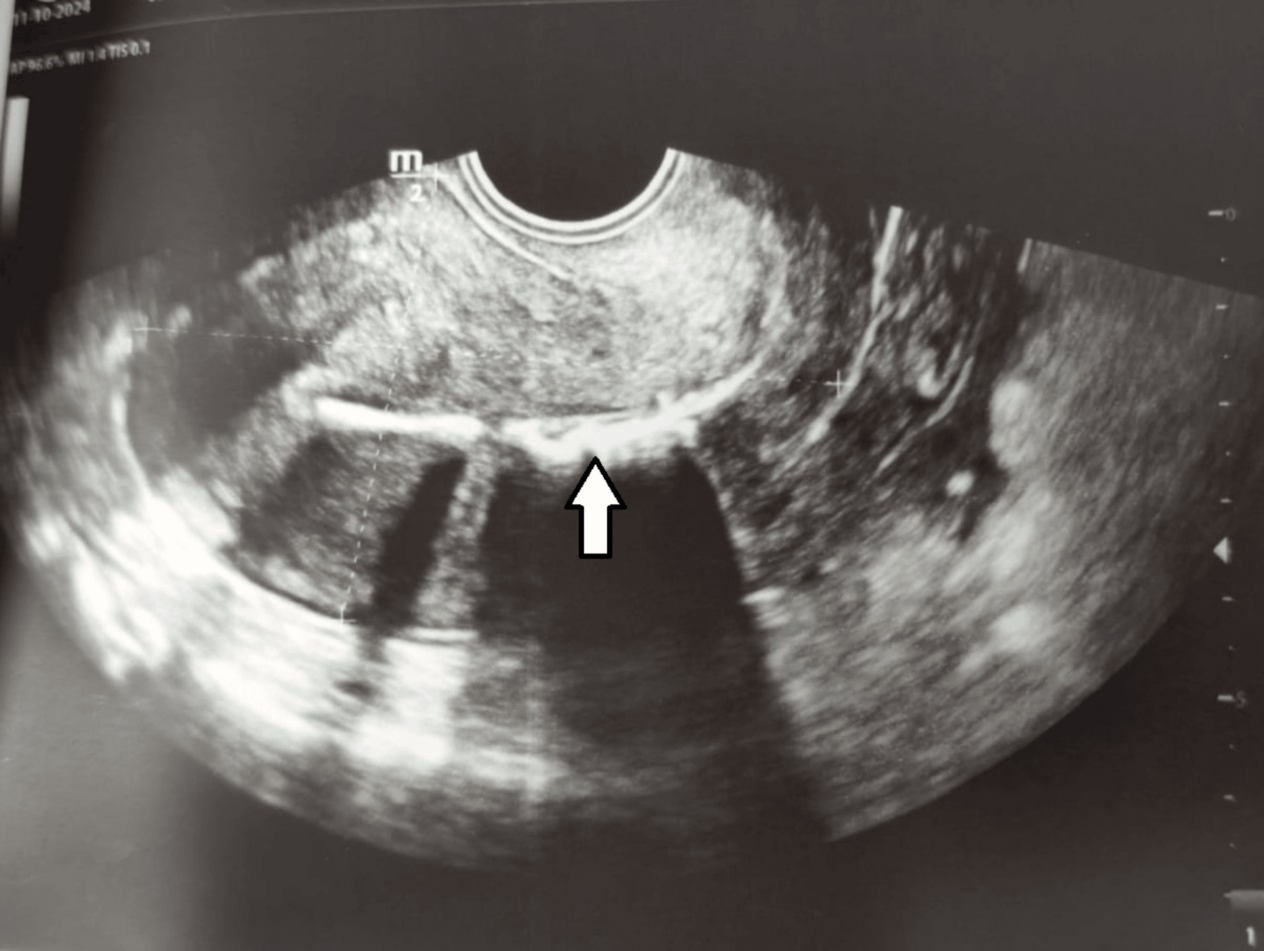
2D transvaginal ultrasound reveals multiple echogenic structures inside the endometrial cavity, the largest measuring 17.5 mm (white arrow).
